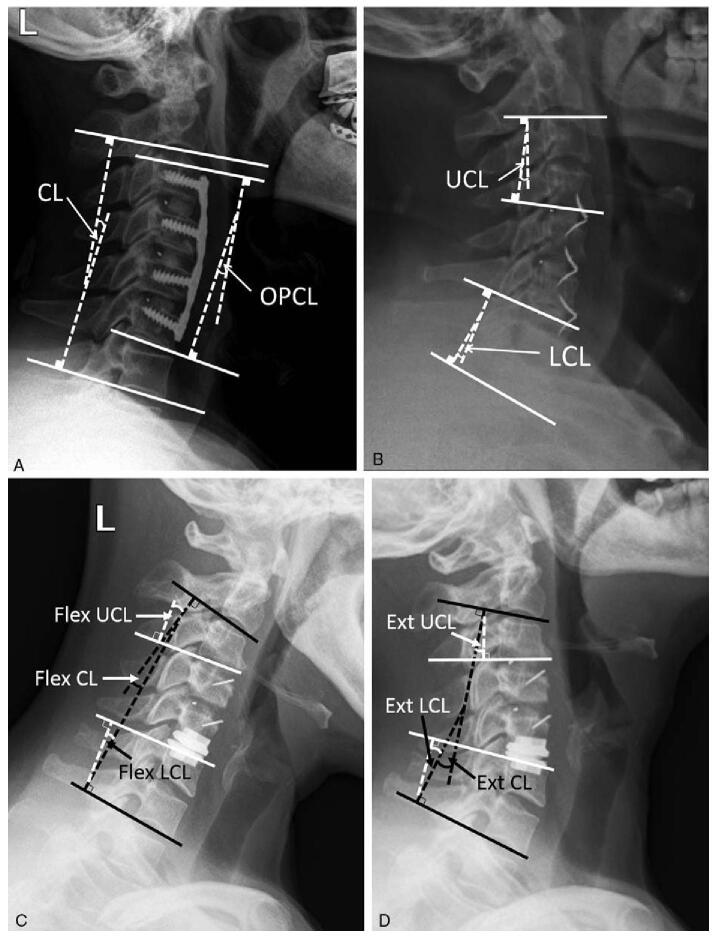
Measurements of cervical alignment on neutral lateral X-ray. (A): The measurements of CL and OPCL; (B): the measurements of UCL and LCL; (C, D): The measurements of UROM and LROM. CL = C2–7 lordosis, LCL = lower adjacent-segment cervical lordosis, LROM = lower adjacent-segment range of motion, OPCL = operated-segment cervical lordosis, UCL = upper adjacent-segment cervical lordosis, UROM = upper adjacent-segment range of motion.
